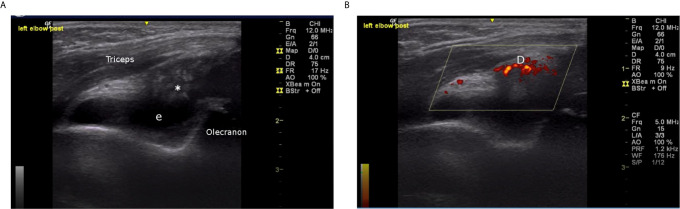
(A) Left elbow, posterior longitudinal view, greyscale (10 year old male, Case 6), demonstrating dense proliferative synovitis (*) in olecranon fossa with effusion (e). (B) Left elbow, posterior longitudinal view, color Doppler (10 year old male, Case 6), demonstrating intense Doppler signal (D) in olecranon fossa.
